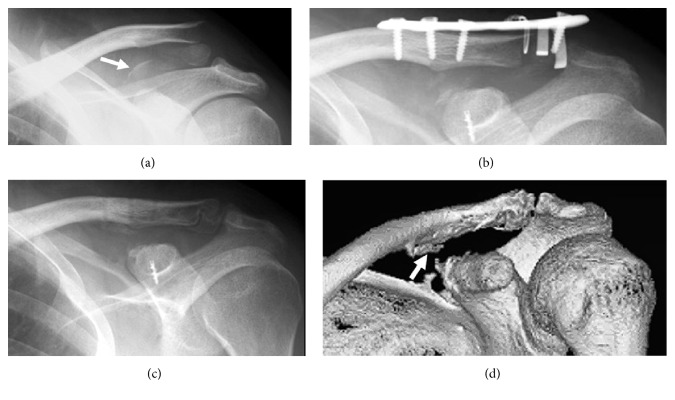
Imaging of Case  1. (a) Radiogram taken at the first visit showing distal clavicular fracture and avulsion fracture of the upper side of the coracoid process (arrow). (b) Postoperative radiogram revealing the well-reduced clavicular fracture and the fractured coracoid fragment. (c) Roentgenogram taken 1 year postoperatively indicating firm bony union of the distal clavicular and coracoid process fractures. (d) Three-dimensional computed tomography performed 1 year postoperatively demonstrating thin new bone formation at the conoid tubercle (arrow).
